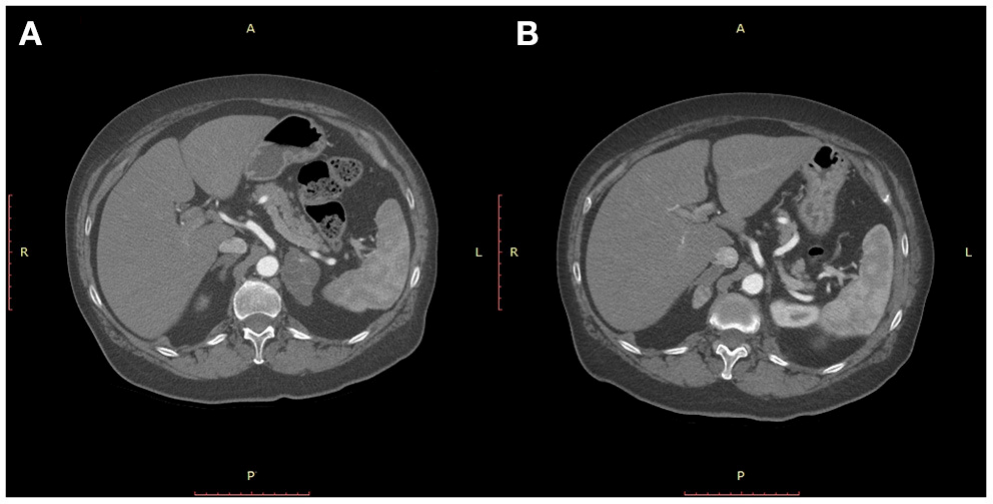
CT-scan of the abdomen showing a 5 cm diameter mass of the left adrenal gland 37 months after a quadrantectomy for an infiltrating ductal carcinoma of the breast (A) and a metachronous 2 cm diameter mass of the right adrenal 15 months after left adrenalectomy (B).
